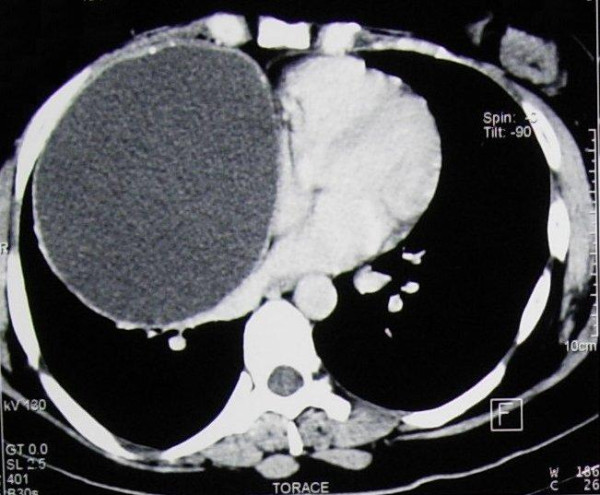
Chest computed tomographic scan revealing the mass compressing the mediastinal vessels.
